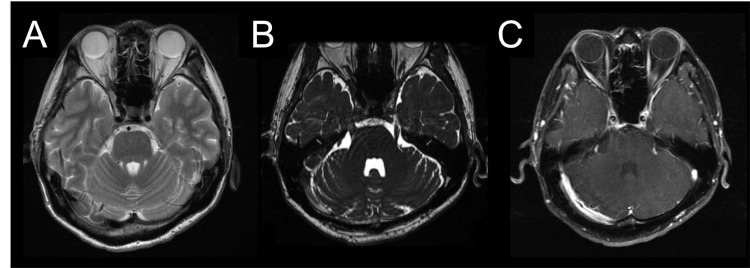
Brain magnetic resonance imaging was performed 44 days after onset. T2-weighted imaging demonstrated the resolution of the hyperintensity in the white matter of the right temporal lobe and pons (A). Fast imaging employing steady-state acquisition sequences revealed a reduction in the size of the right trigeminal nerve (B), and contrast-enhanced T1-weighted imaging showed no enhancement of the right trigeminal nerve (C).
